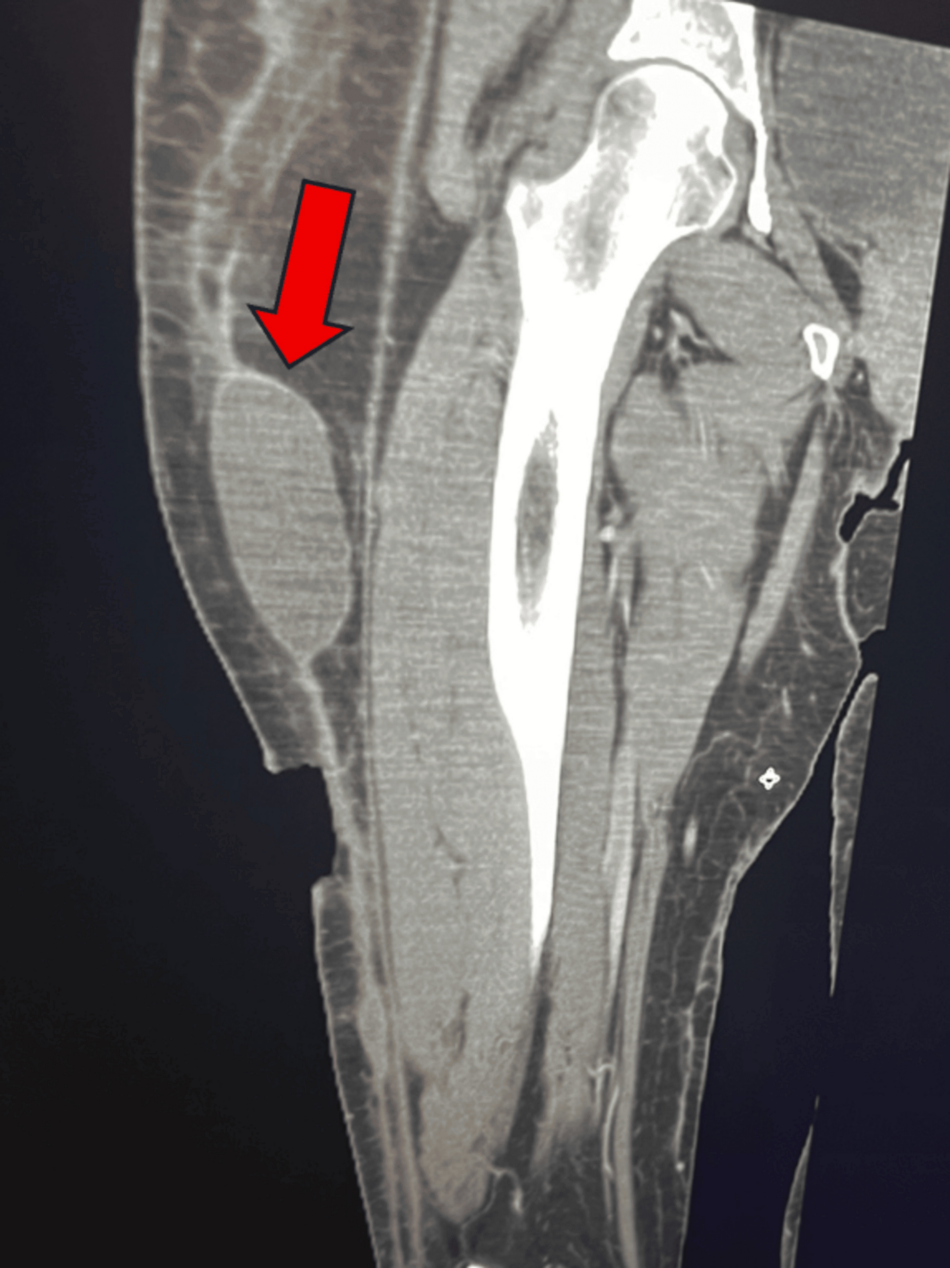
Extensive Subcutaneous Fluid Collection of the Right Anterior Thigh Consistent With Suspected Morel-Lavallée Lesion Axial computed tomography (CT) image of the right thigh demonstrating a large, attenuated fluid collection within the subcutaneous adipose tissue involving the entirety of the anterior thigh (red arrow). Imaging was obtained to evaluate for a suspected Morel-Lavallée lesion and/or underlying abscess following progression of an anterior thigh wound to eschar formation.
